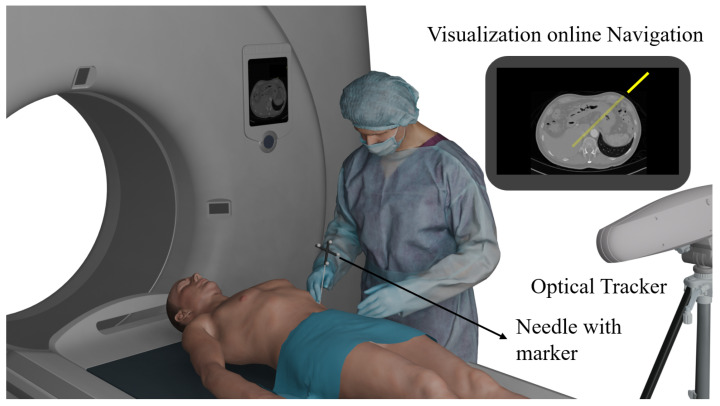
Example of a CT-guided needle biopsy procedure assisted by a stereoscopic IR optical tracking system, enabling real-time registration and visualization of the needle within the tomographic images.
